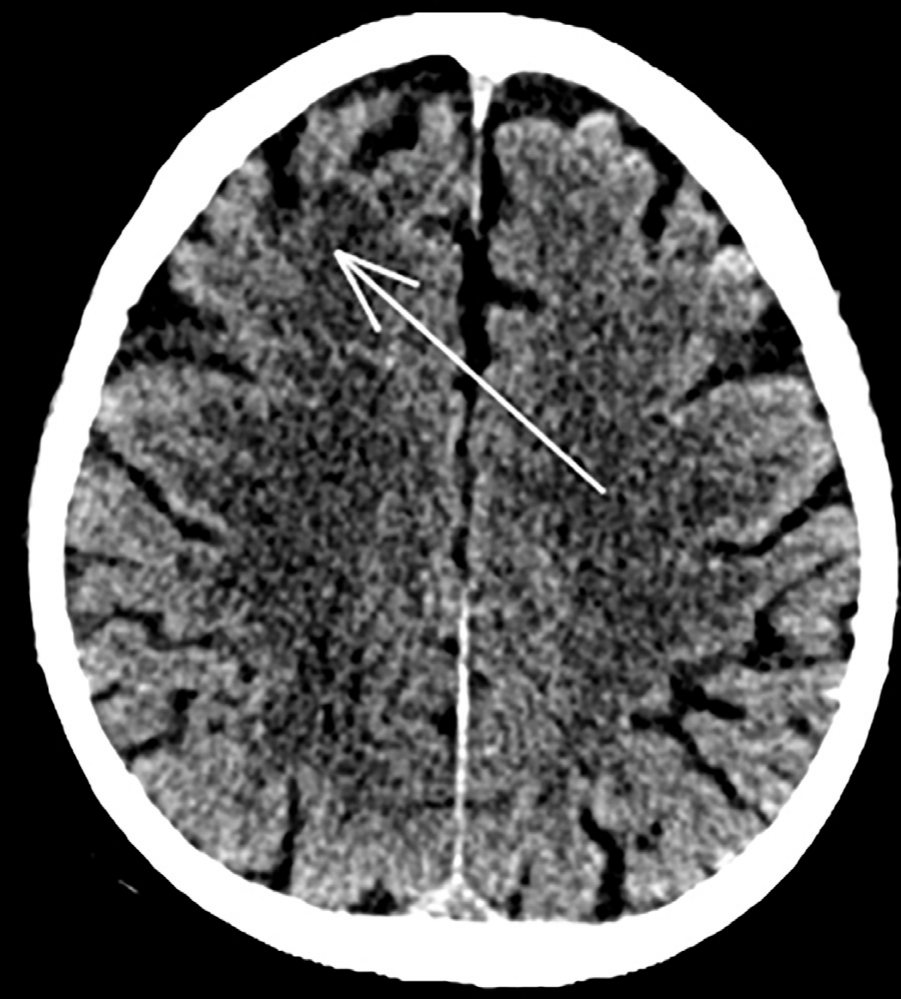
CT showing a small hypodensity (white arrow) in the right upper frontal cortico-subcortical region due to ischemic lesion.
